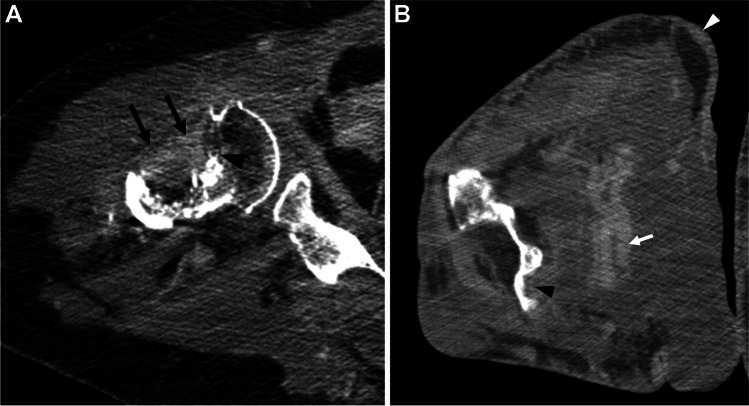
CT axial image at the level of the humeral head (A) shows area of cortical destruction (black arrowhead) with enhancing soft tissue (black arrows). At the level of the elbow (B), there are skin thickening (white arrowhead), tortuous blood vessels (white arrow), and erosion of the medial humeral condyle (black arrowhead)
